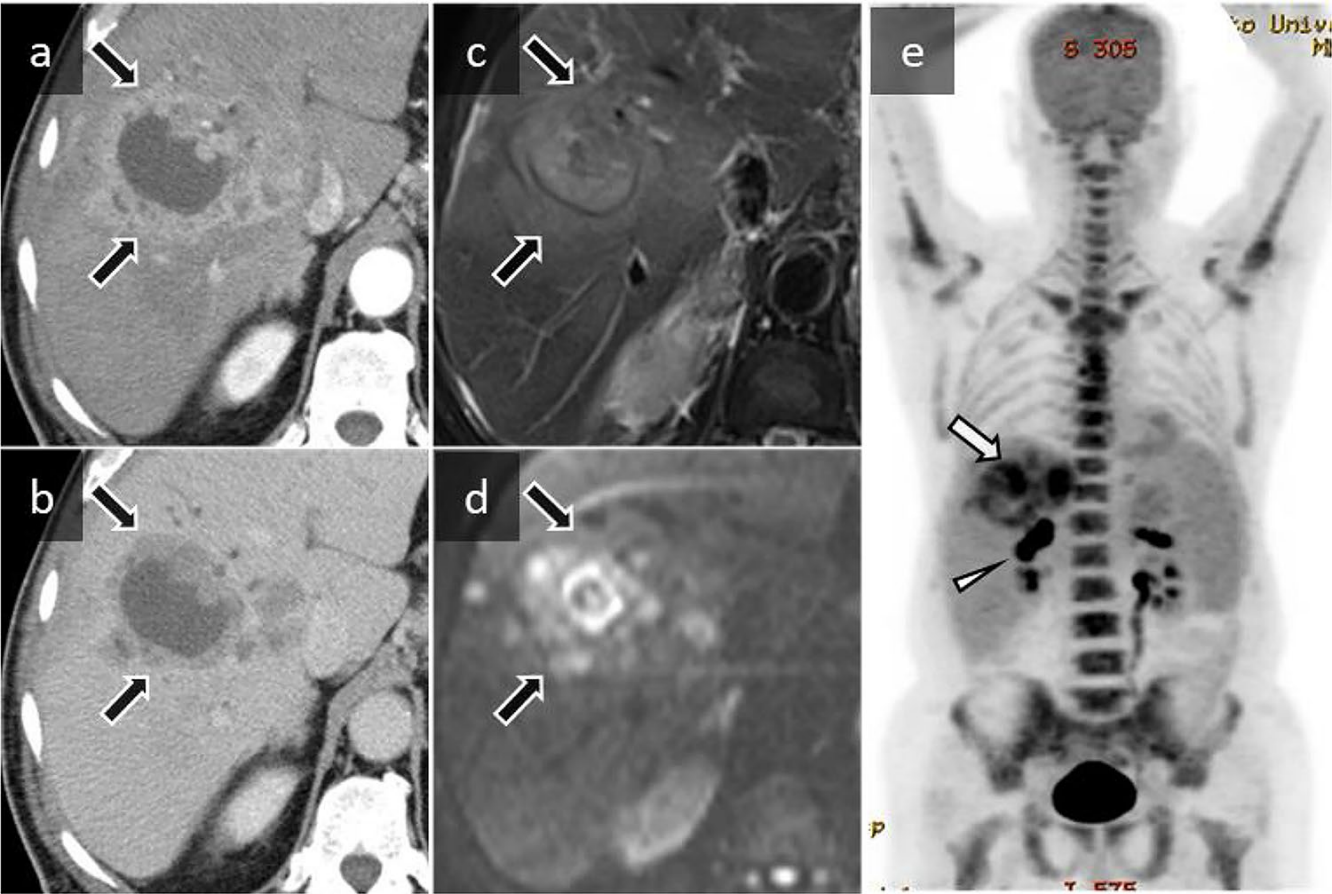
A 62-year-old man with G-CSF-producing hepatocellular carcinoma. a, b Axial contrast-enhanced CT image shows multiple rounded masses in the right lobe of the liver with geographic hypoattenuation and enhancement in the peripheral component, which are enhanced in the early phase and washed out in the late phase (black arrows). c, d Axial fat-suppressed turbo spin echo T2-weighted and diffusion-weighted imaging at b = 1000 s/mm^2^ shows that the signal intensity of the masses is mild hyperintensity relative to liver. e An ^18^F-FDG-PET/CT maximum intensity projection image demonstrates FDG uptake in the hepatic tumor (maximum standardized uptake value = 12.2, white arrow) and lymph node metastasis (white arrowhead) and diffuse uptake in the bone marrow of the spine, pelvis, and proximal metaphysis of the long bones. The splenic uptake is slightly more intense than the hepatic uptake
